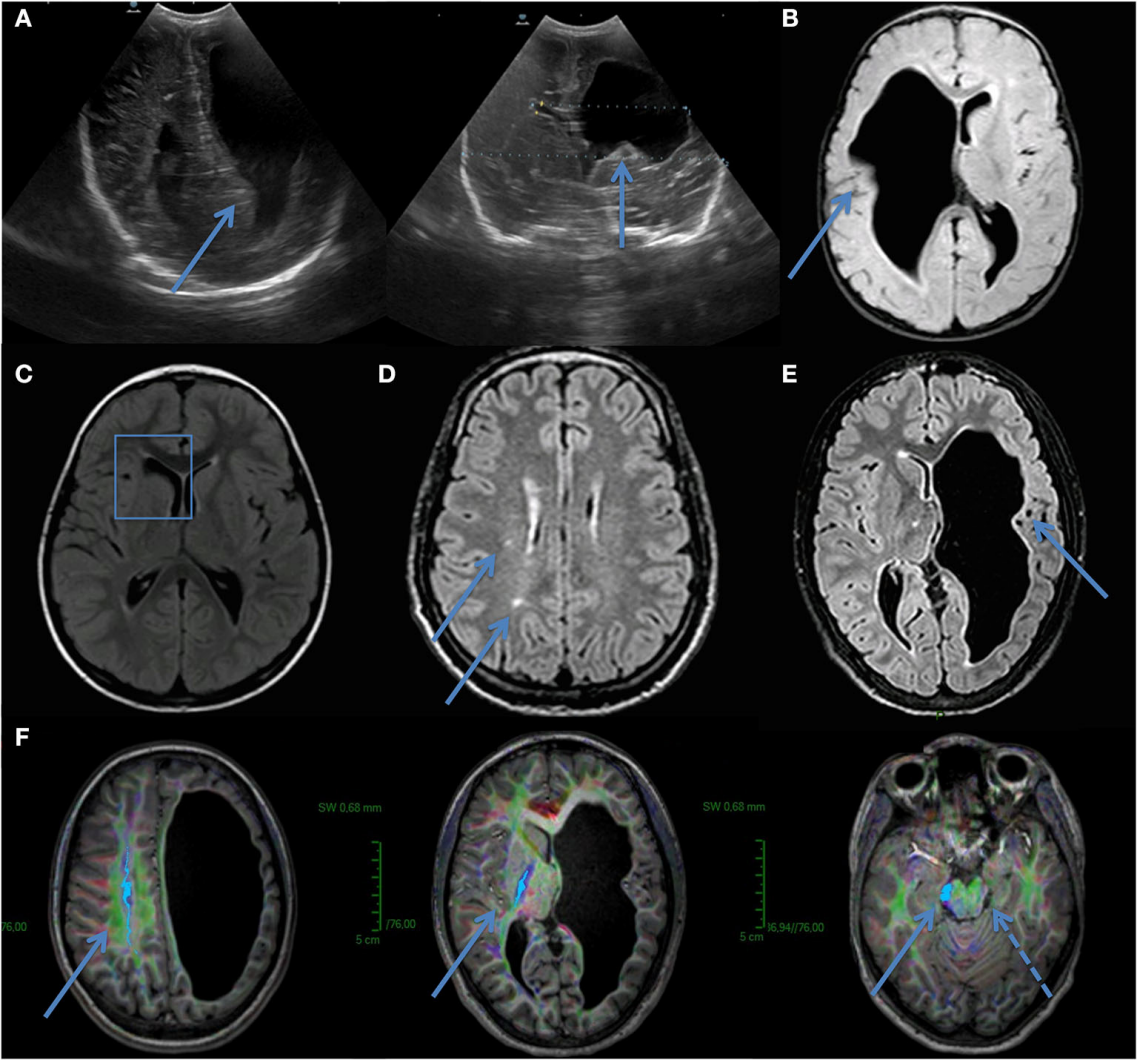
Ultrasound *in utero* from IV-6 (A). Image showed ventricular asymmetry and brain MRI confirmed right frontotemporal dilatation (B). IV-5–Brain MRI revealing porencephalic cyst of frontal horn of lateral right ventricle (C). (D) III- 3–Brain MRI showed small asymptomatic lesions in white matter. No microbleeds or cystic cavities were found. (E,F) IV-3–Brain MRI showed left frontotemporal dilatation and diffusion tensor imaging (DTI) sequences demonstrated no left corticospinal tract (cranio-caudal fibers, indigo, with arrows). A dashed arrow indicates secondary atrophy in the left cerebral peduncle.
